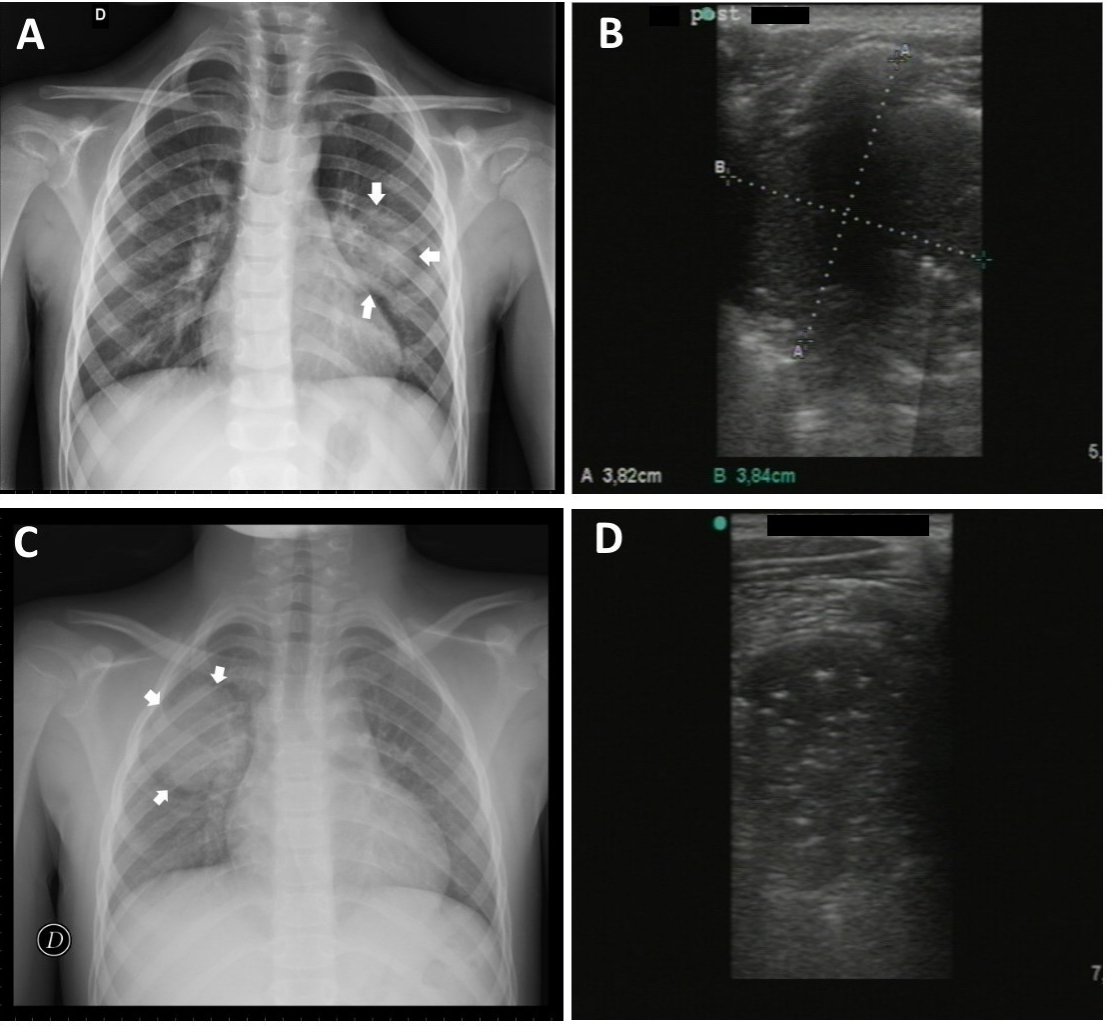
Round pneumonia. Case 1. 5 year old male with evidence of round pneumonia by chest X-ray in middle region of the left lung (A) duly detected by lung ultrasound (B) Case 2. 8 year old male with round pneumonia in middle/upper region of right lung by chest rx (C) and corresponding ultrasound image (D).
